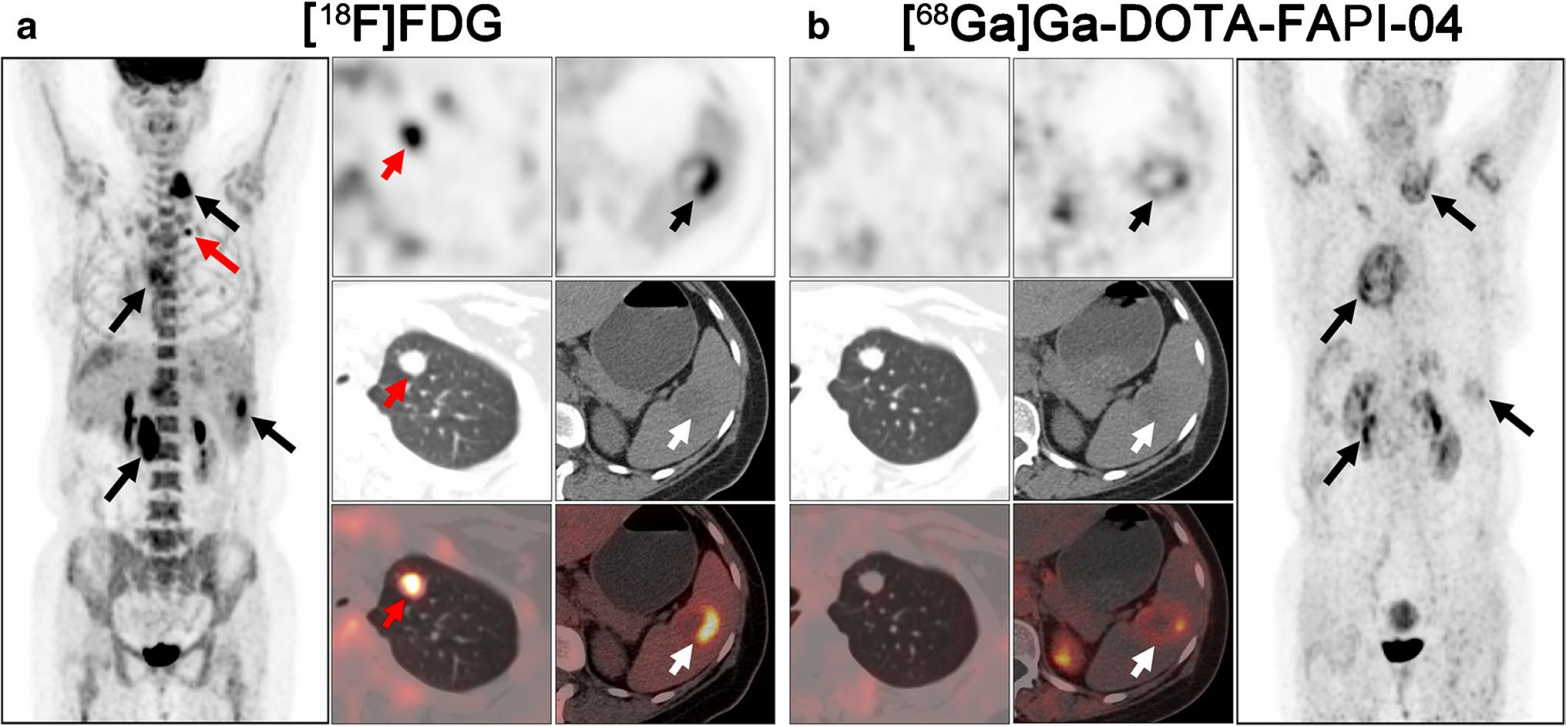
A 44-year-old woman (patient #44) pathologically confirmed with liver follicular dendritic cell sarcoma (FDCS) received radical operation 6 months ago. [^18^F]FDG PET/CT (a) demonstrated all the metastatic lesions with intense [^18^F]FDG activity, including liver, spleen, lung, and lymph node metastases. Compared with [^18^F]FDG, [^68^ Ga]Ga-DOTA-FAPI-04 PET/CT (b) demonstrated the liver, spleen, and lymph node metastases with moderate [^68^ Ga]Ga-DOTA-FAPI-04 activity. But no intensive [^68^ Ga]Ga-DOTA-FAPI-04 uptake was observed on the lung metastatic lesions. Black and white arrows indicated the tumor lesions detected by both tracers, and red arrows indicated the tumor lesions detected by [^18^F]FDG alone
